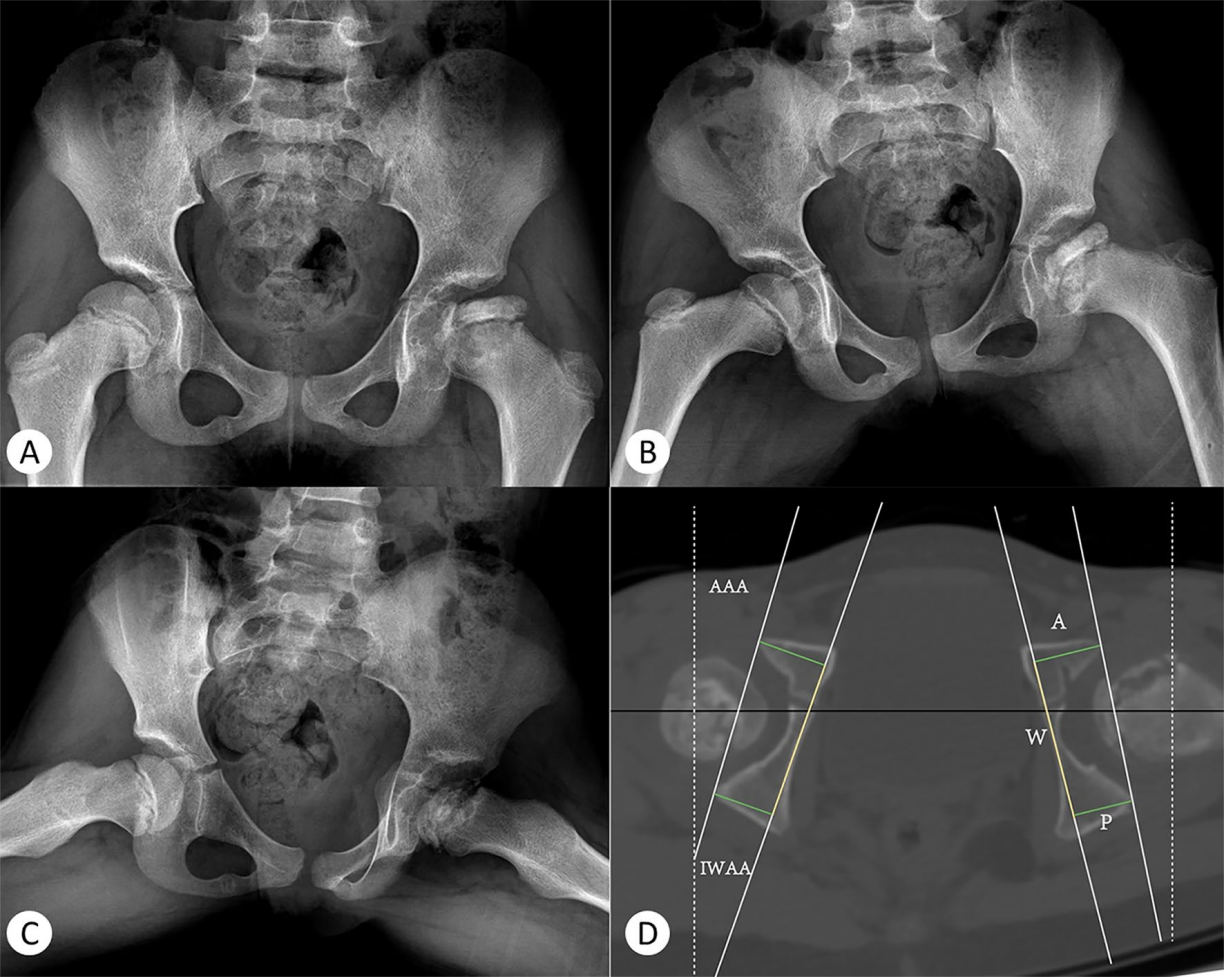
An eight-year-old girl with Legg–Calve–Perthes disease in the left hip. The anteroposterior pelvis radiograph (A), Von-Rosen pelvis radiograph (B) and frog pelvis radiograph (C) show that patient was in Waldenström II stage, Herring B stage and Catterall III stage.
